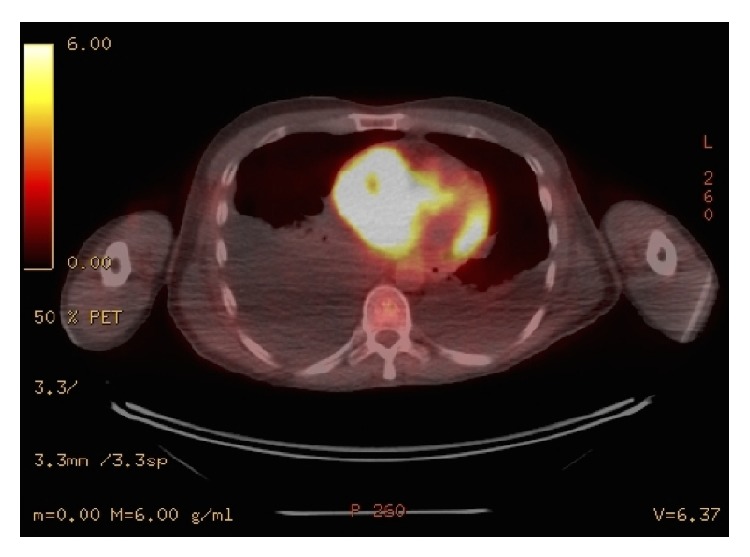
PET/CT scan displaying metastatic masses in right atrium and liver, as well as bilateral pleural effusion due to heart failure.
